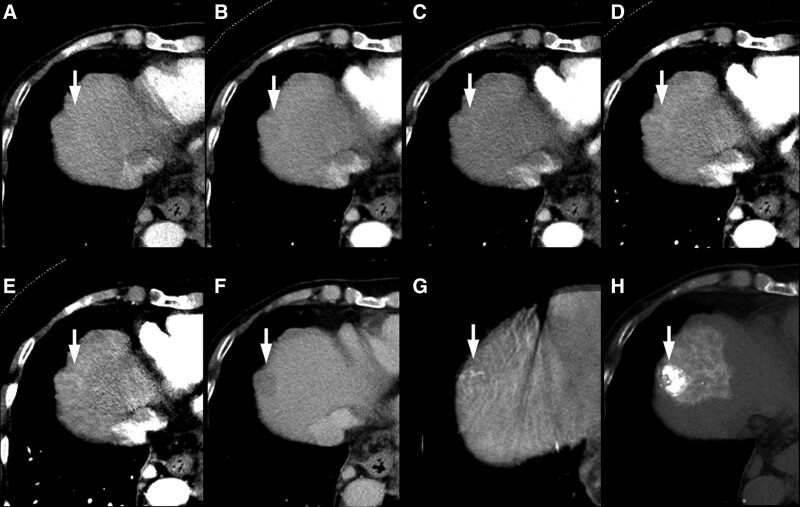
An 84-year-old female patient with slightly hypervascular small HCC. HAP images with (A) 140 kVp, (B) blended 120 kVp, and (C) 80 kVp and VMI at (D) 50 keV and (E) 40 keV, (F) DP SE-CT image, (G) angiographic CT-like image, and (H) post-transcatheter arterial chemoembolization therapy iodized-oil CT image are shown. Sensitivity scores for early HCC enhancement were 1, 1, 3, 4, and 5 in order from (A) to (E), respectively, and the hypervascularity was only detectable with low keV VMI images. DP = delayed phase, HAP = hepatic arterial phase, HCC = hepatocellular carcinoma, SE-CT = single-energy CT, VMI = virtual monochromatic imaging.
